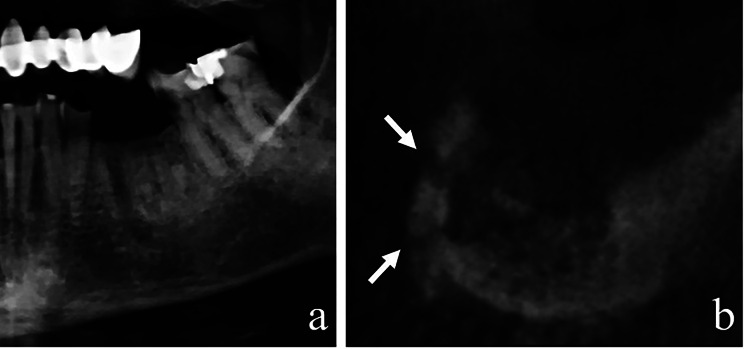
(a) A cropped PR image showing osteonecrosis in the mandibular posterior region. (b) A cropped coronal CBCT image showing a pathologic fracture in the buccal cortical wall of the mandible (white arrows)
